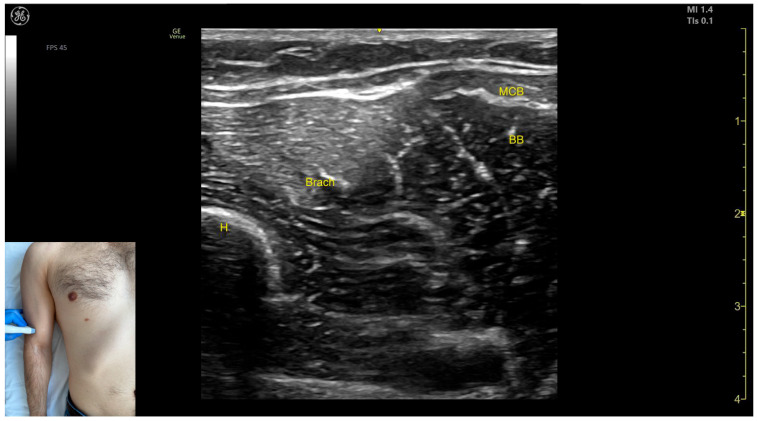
Ultrasound anatomy of the brachialis (Brach) with key landmarks: Brach—brachialis; H—humerus; MCB—musculocutaneous branch; and BB—bicep brachii.
